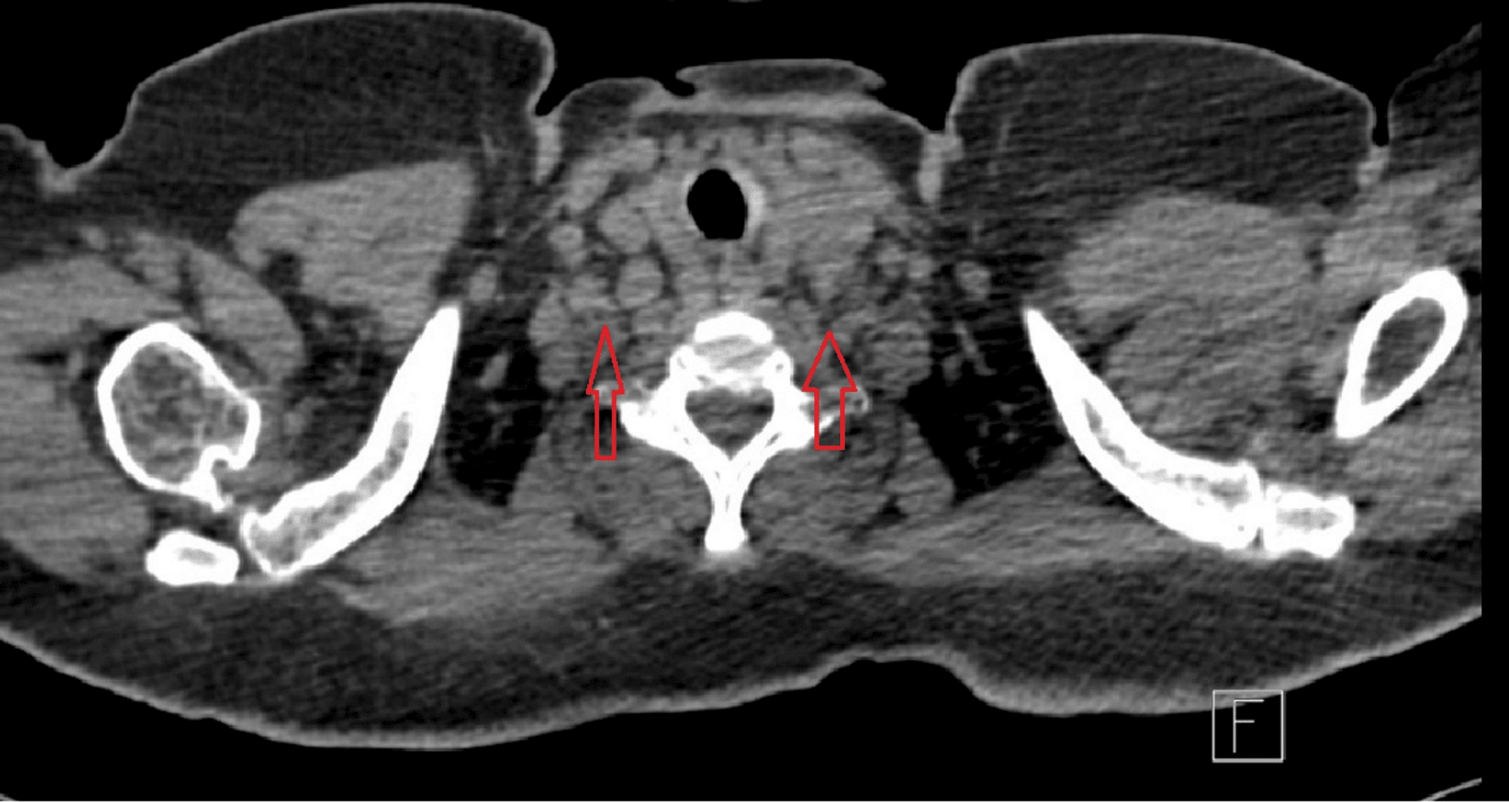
Lower neck cuts of a high-resolution CT scan of the chest showing multiple enlarged lymph nodes bilaterally
